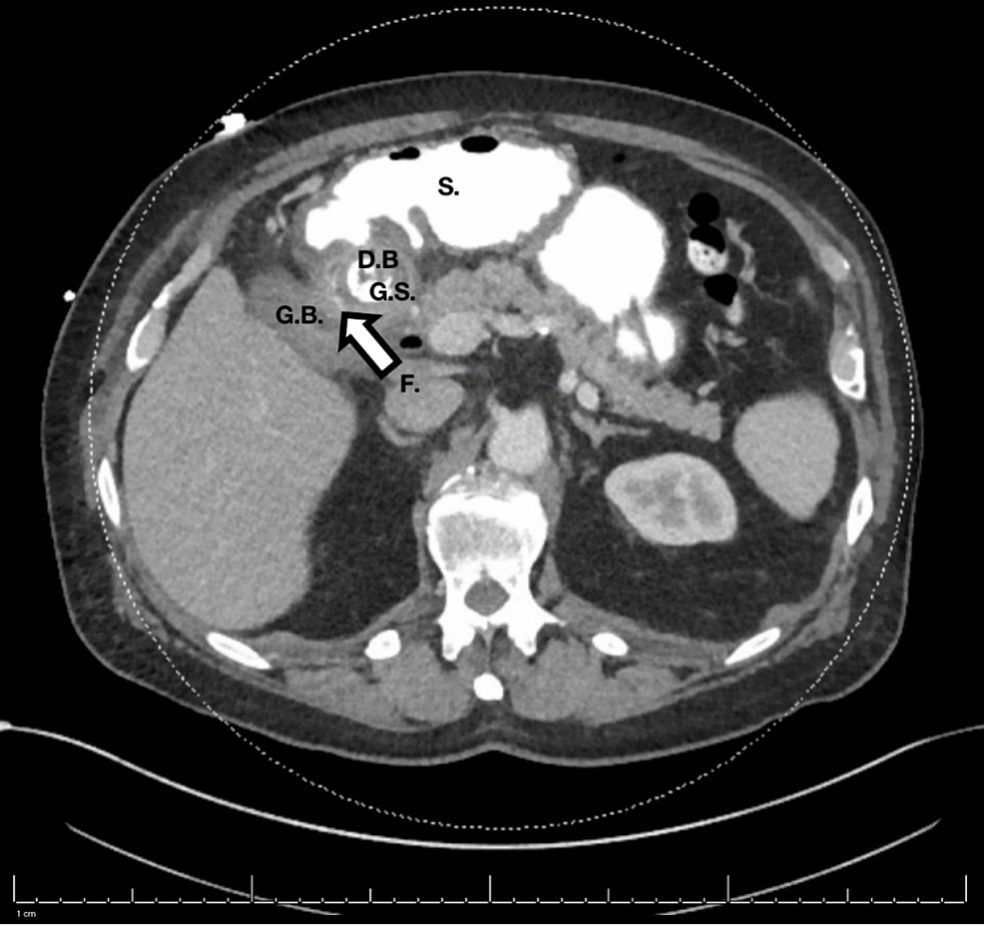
Computed tomography (CT) scan of the abdomen. A large gallstone (G.S.) was seen in the duodenal bulb (D.B) lumen adjacent to the gallbladder (G.B.), indicating a cholecystoduodenal fistula (F., arrow). Anterior to the duodenum is the stomach (S.), which is filled with contrast.
